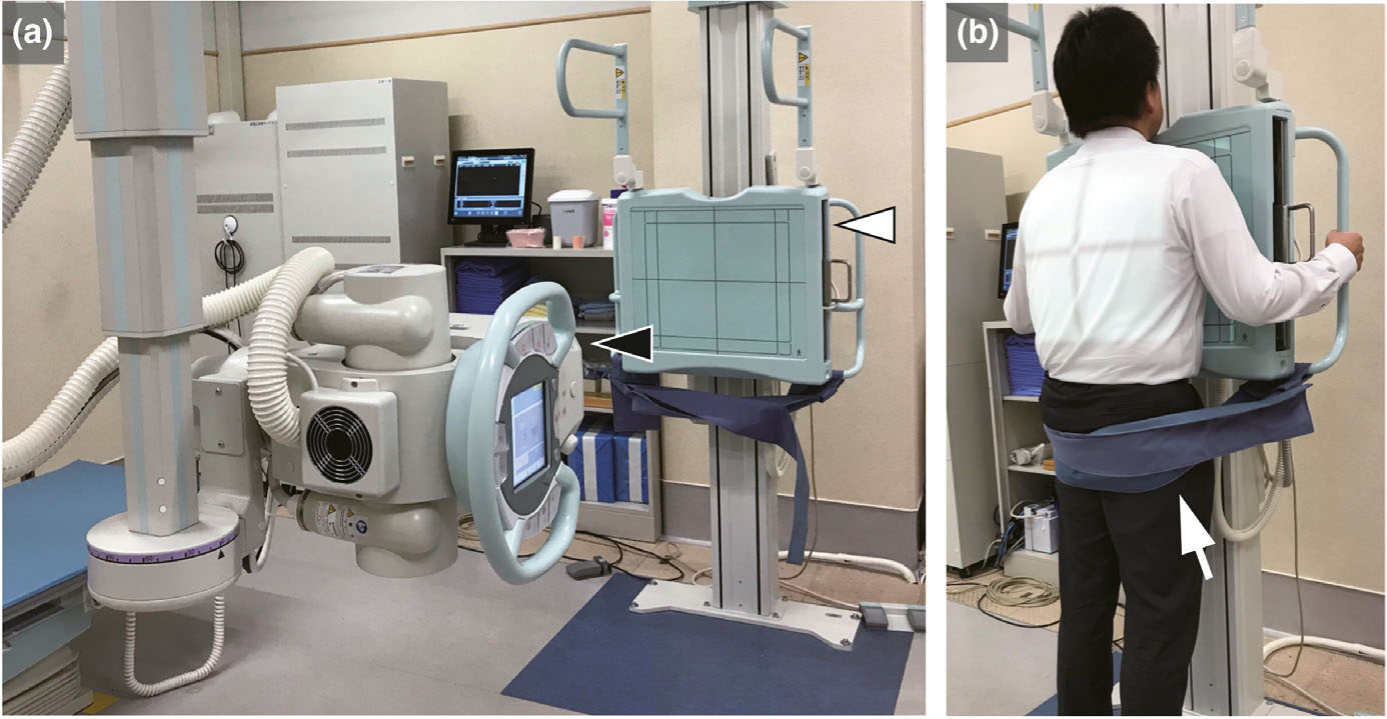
Clinical practice of chest digital dynamic radiography (DDR). (a) Flat panel detector system (Konica Minolta, Inc., Tokyo, Japan) was composed of a flat panel detector (AeroDR fine; Konica Minolta, Inc., Tokyo, Japan) (white arrowhead) and a pulsed x‐ray generator (RAD speed Pro; Shimadzu Corporation, Kyoto, Japan) (black arrowhead). In addition to chest DDR, conventional chest radiographs can also be taken. (b) Standing posteroanterior position in chest digital dynamic radiography. In order to minimize motion artifact, the pelvis is fastened firmly by a belt (white arrow).
